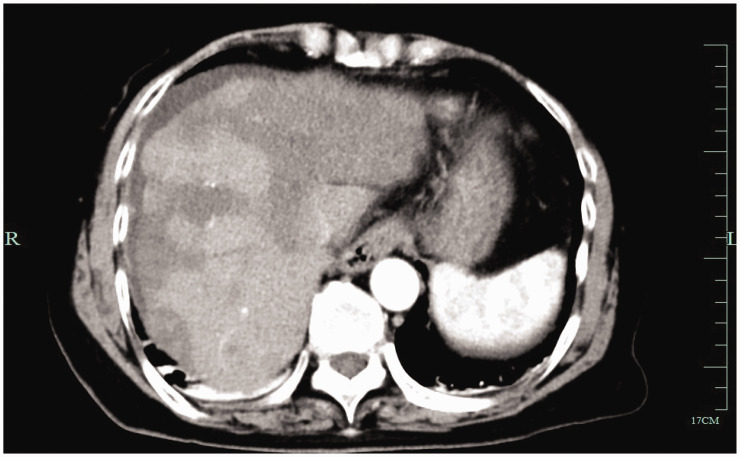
Enhanced computed tomography showing recurrent intrahepatic metastasis of the tumor at a 5-year follow-up.
